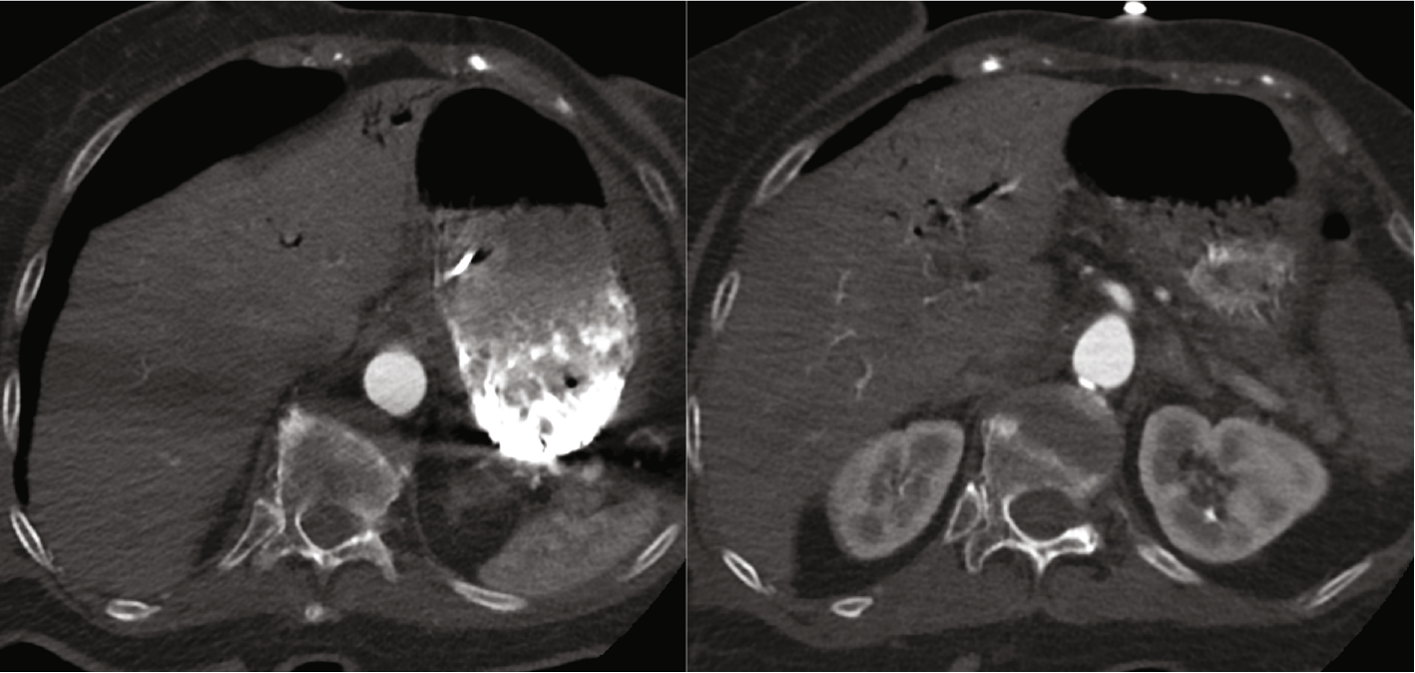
Axial CT images of the upper abdomen demonstrate portal venous gas in the liver hilum and left hepatic lobe.
